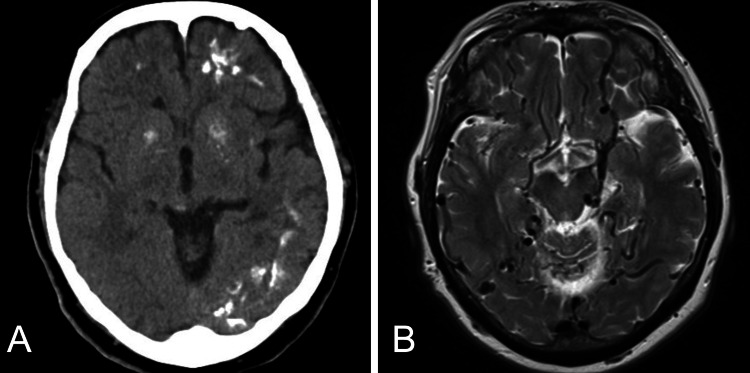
Preoperative imagings (A) Computed tomography revealing multiple calcified lesions in the left cerebral hemisphere. (B) Magnetic resonance imaging showing vein dilatation in the whole cerebrum
